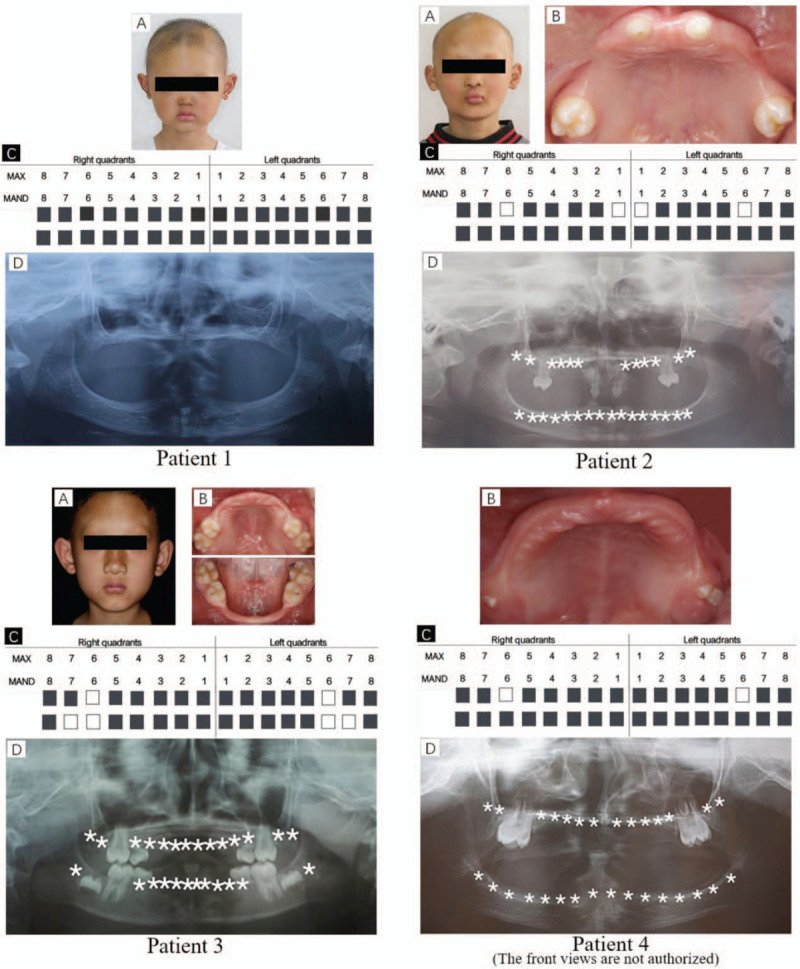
Characteristics of individuals with hypohidrotic ectodermal dysplasia (HED). (A) Positive image of probands; (B) Intraoral image of probands; (C) Legend of intraoral missing teeth of probands; (D) Panoramic radiography of probands.
